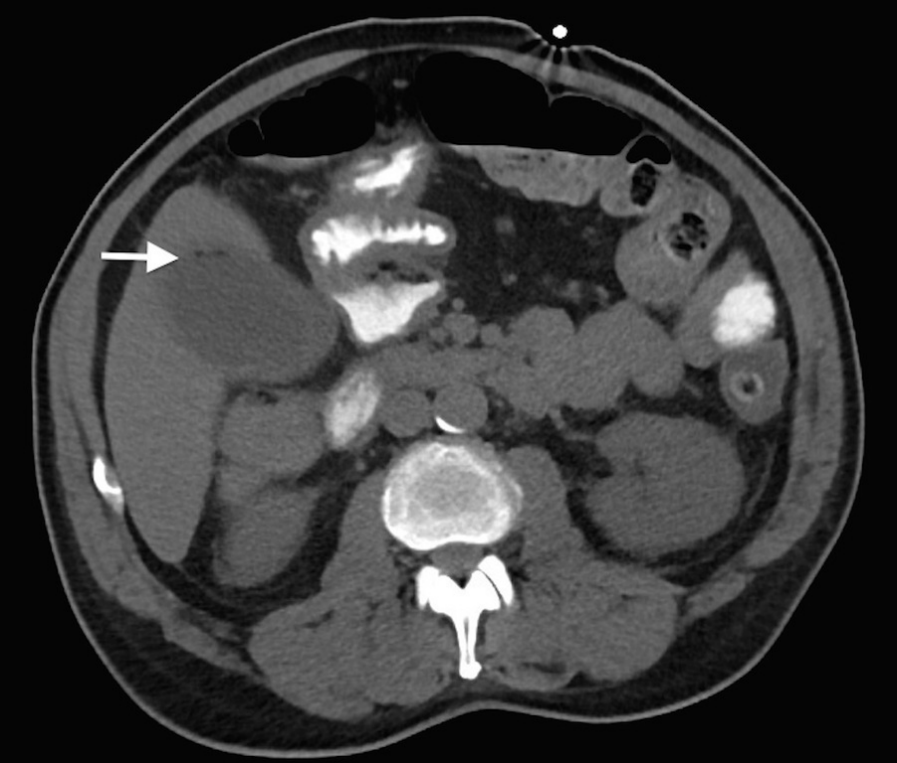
Image showing the computed tomography (CT) of the abdomen. Axial computed tomography (CT) image demonstrating collections of gas near the anterior gall bladder wall (white arrow).
